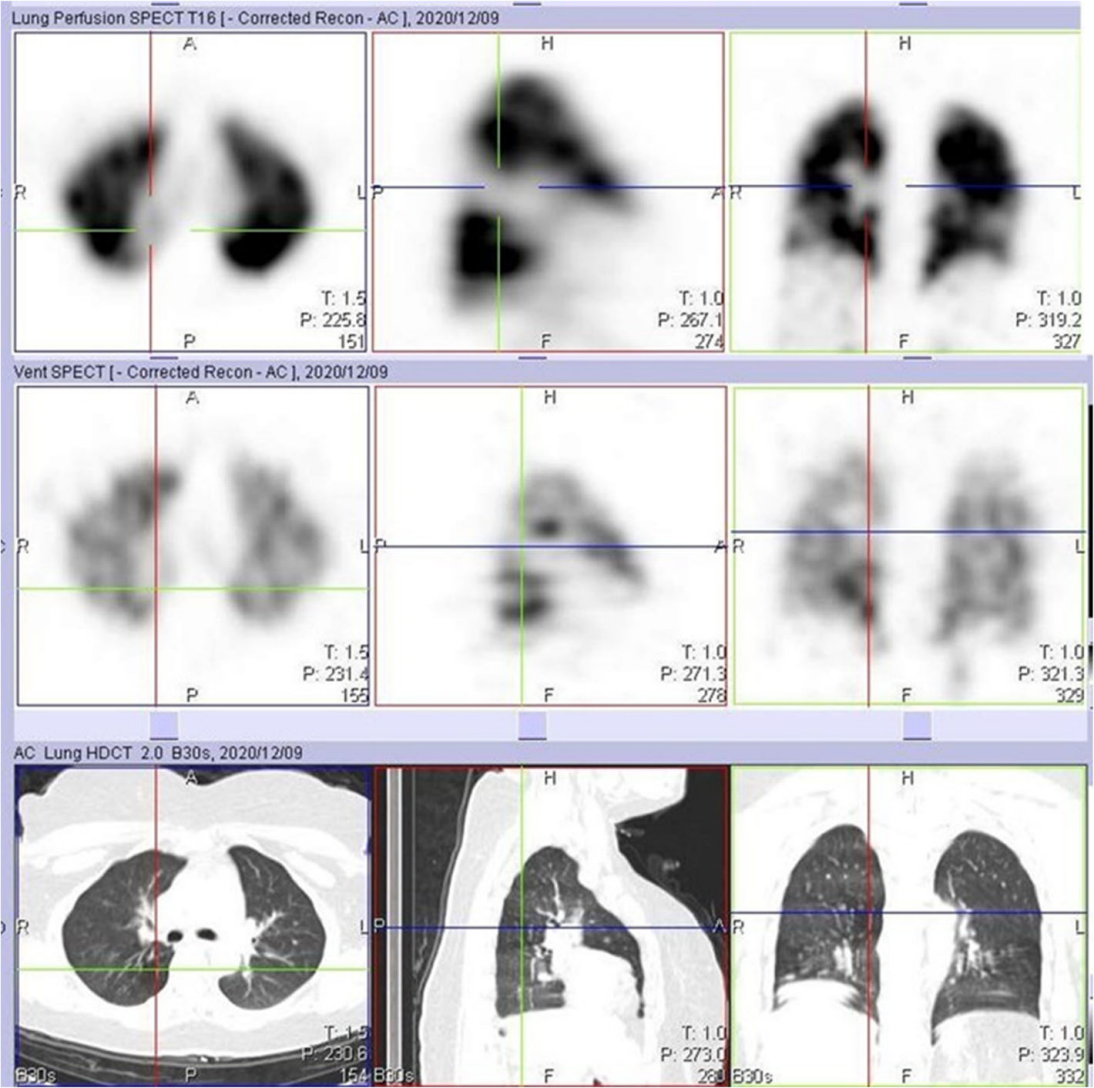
Ventilation perfusion SPECT/CT study of the same case in Fig. 7 performed 3 months after initiating anticoagulation showing matching, with no reduction in the size of the perfusion defect. Note the two unmatched perfusion defects in both lower lung zones
